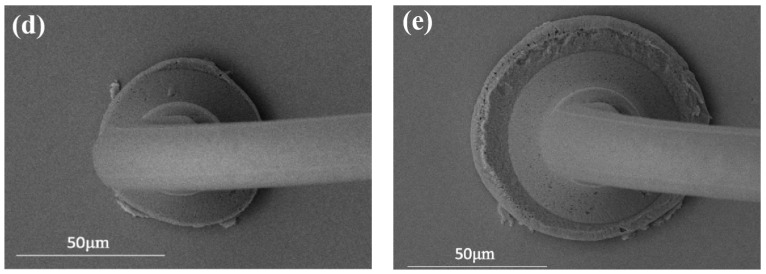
Morphology of Pd80 ball-bonded points under different bonding pressure/ultrasonic power. ((a) 40 g/110 mW; (b) 40 g/100 mW; (c) 40 g/120 mW; (d) 35 g/110 mW; (e) 45 g/110 mW).
